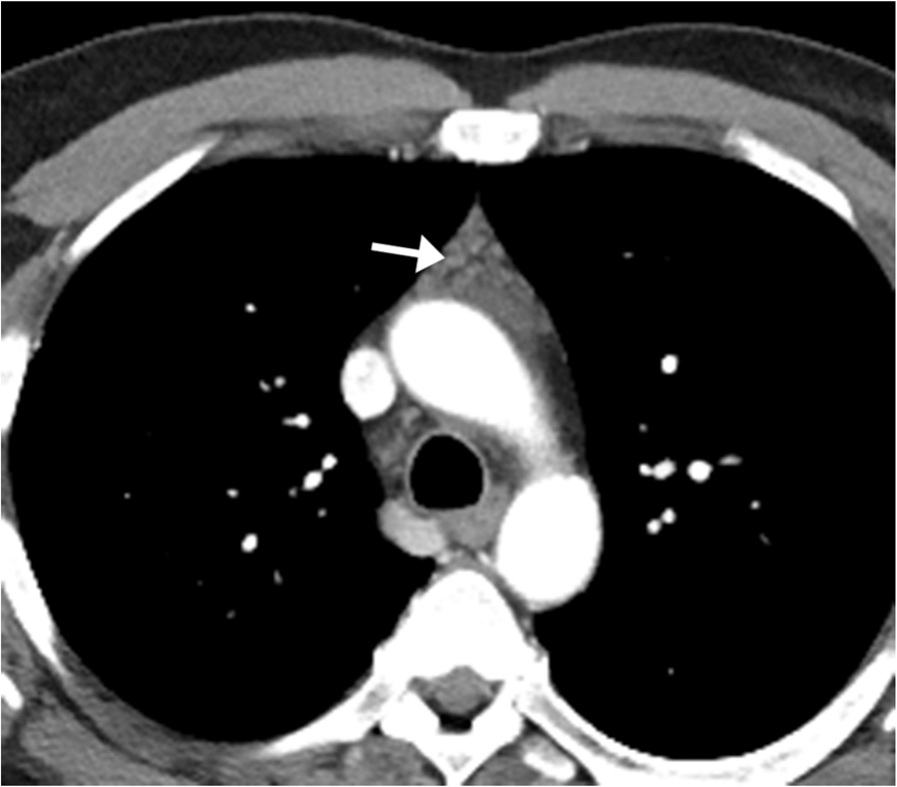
Thymic hyperplasia. Axial CT image of a 60-year-old patient undergoing chemotherapy demonstrates an anterior mediastinal mass (arrow) with the expected location, configuration, and attenuation of the thymus gland, consistent with rebound thymic hyperplasia secondary to chemotherapy. It is important to distinguish thymic hyperplasia from thymoma, which can present as asymmetry of the lobes, focal contour abnormality, and demonstrate focal increased FDG uptake
